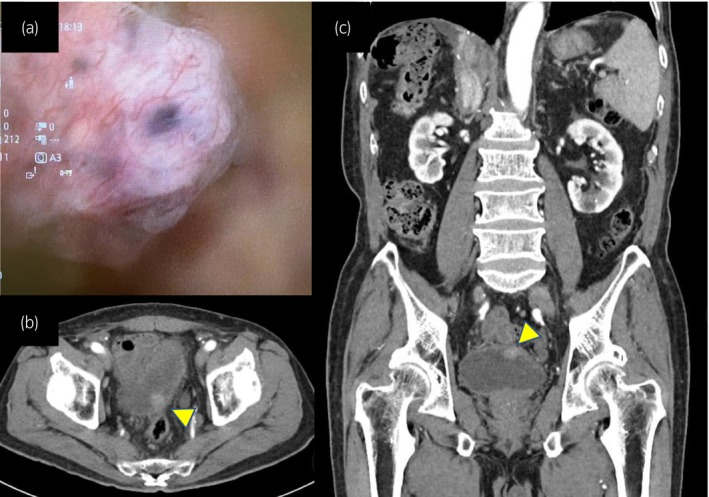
(a) Cystoscopy revealed a solitary submucosal mass on the posterior wall. (b, c) CT showing a 15 mm soft tissue mass protruding from the posterior bladder wall.
